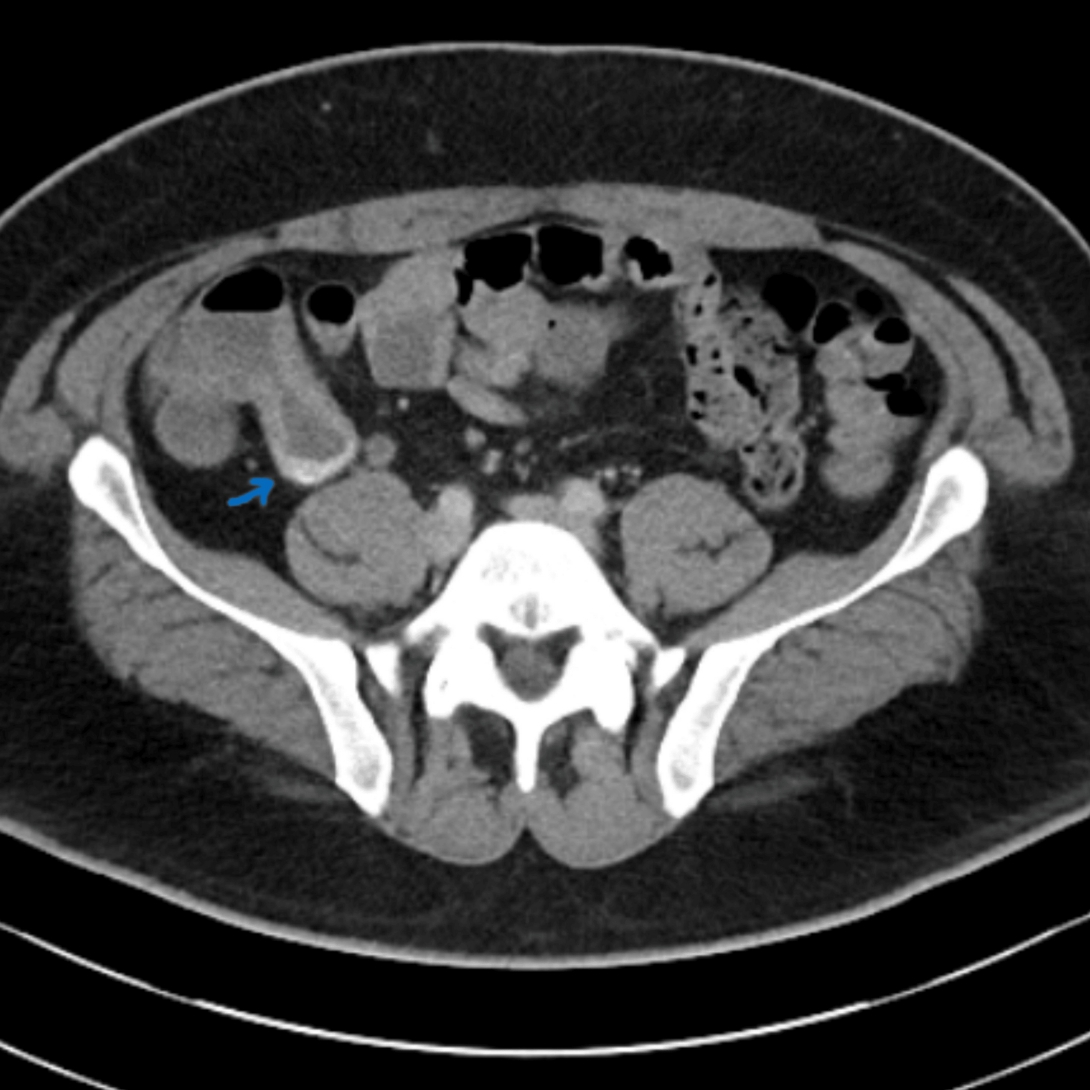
Venous-phase CT angiogram showing prominent contrast extravasation within the thickened appendix, indicative of active bleeding. CT, computed tomography
